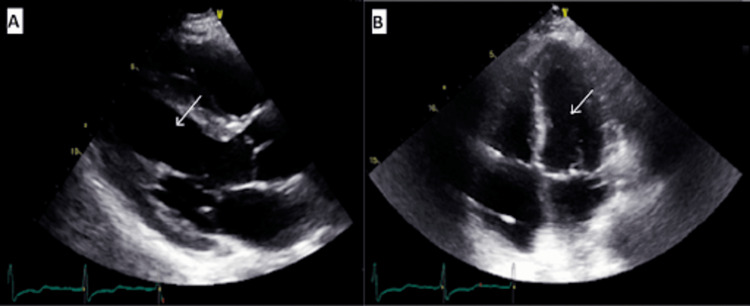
Repeat transthoracic echo two weeks after presentation Parasternal long axis (A) and four-chamber (B) views show a left ventricle (white arrow) ejection fraction of 60% with normal wall motion.
